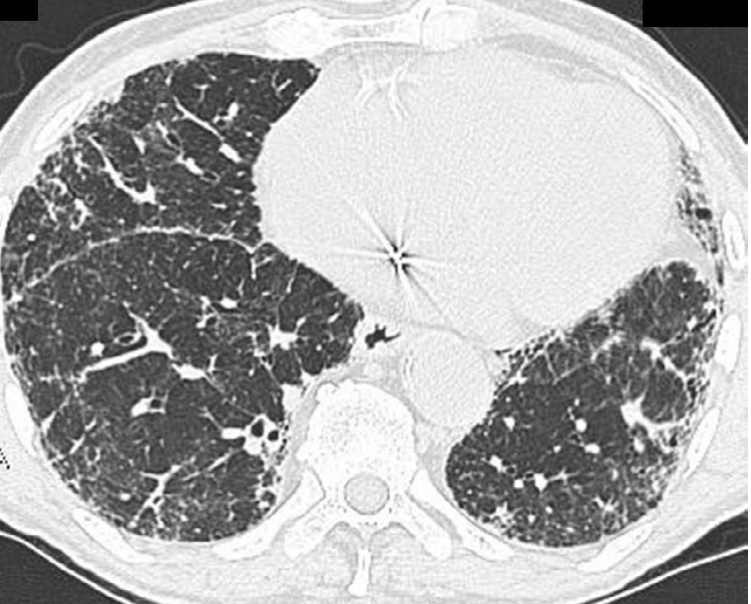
Computed tomography scan of an 81-year old man with biopsy-proven IPF. A peripheral distribution of reticular opacities is demonstrated. Honeycombing and traction bronchiectasis are notably absent. In the absence of specific findings, a surgical lung biopsy was needed to make a diagnosis. * Reprinted from Fishman's Pulmonary Diseases and Disorders, 4^th ^edition 2007. Meltzer, EB and Noble, PW: Chapter 70, Idiopathic Pulmonary Fibrosis. With permission from McGraw-Hill Companies.
